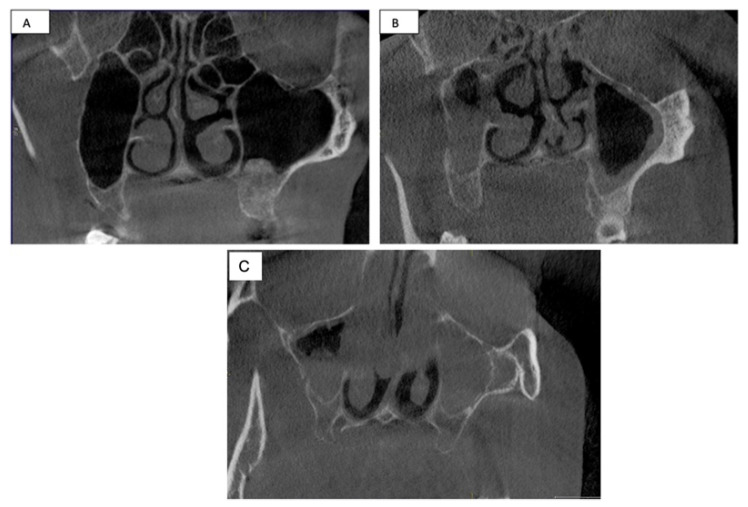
Measurement of CRS via Lund–Mackay staging system. (A) Coronal section of CBCT shows a score 0 for both right and left maxillary sinuses. (B) Coronal section of CBCT shows a score 1 (partial opacification) for both right and left maxillary sinuses. (C) Coronal section of the CBCT shows score 1 in the right maxillary sinus and score 2 (complete opacification) in the left maxillary sinus.
